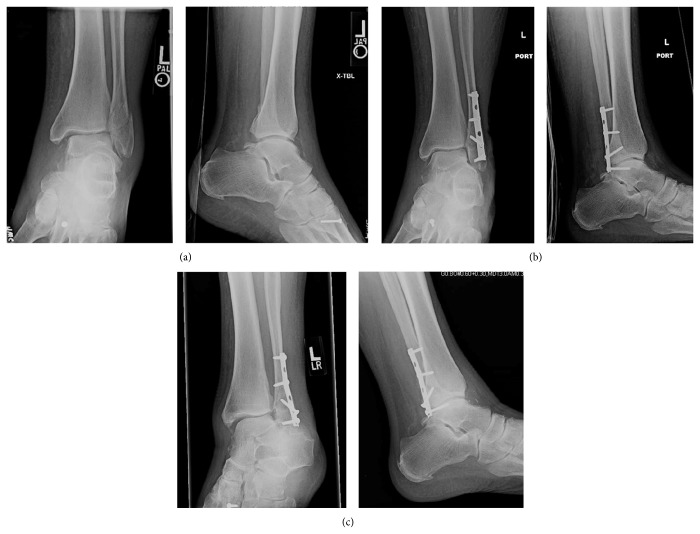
Single case of loss of reduction, suspect secondary to missed syndesmotic injury. (a) Preoperative mortise and lateral radiographs. (b) Immediate postoperative mortise and lateral radiographs. (c) 6 weeks of followup mortise and lateral radiographs.
